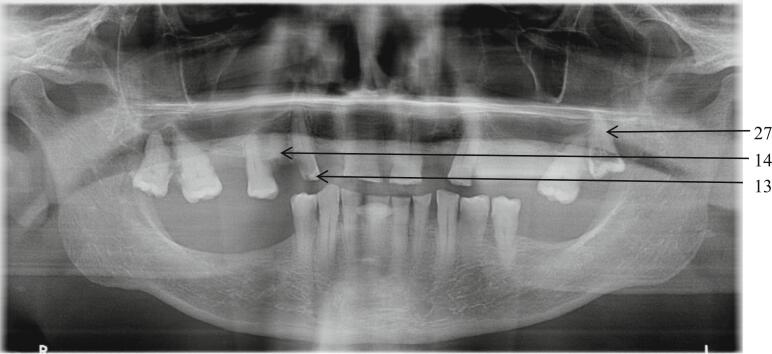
Orthopantomogram, indication for extraction of teeth n°13-14-27.
